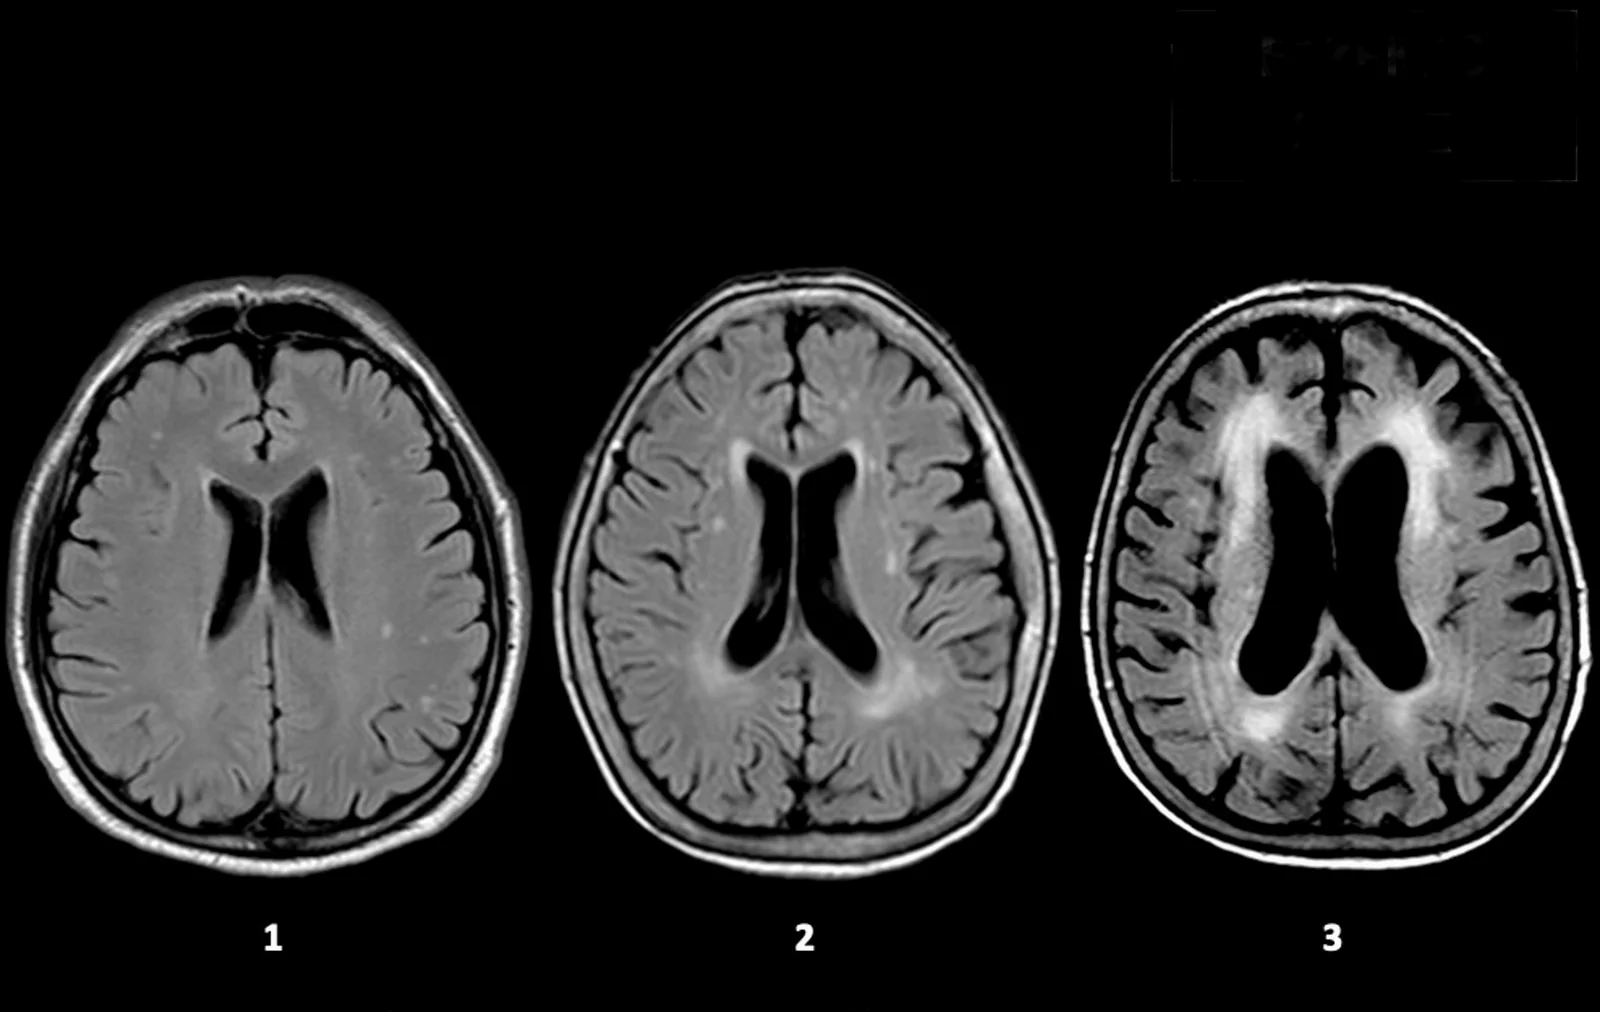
Fazekas score was performed on the T2-FLAIR sequence, and gliotic lesions show a tendency to coalesce, from 1 to 3. FLAIR: fluid-attenuated inversion recovery.
